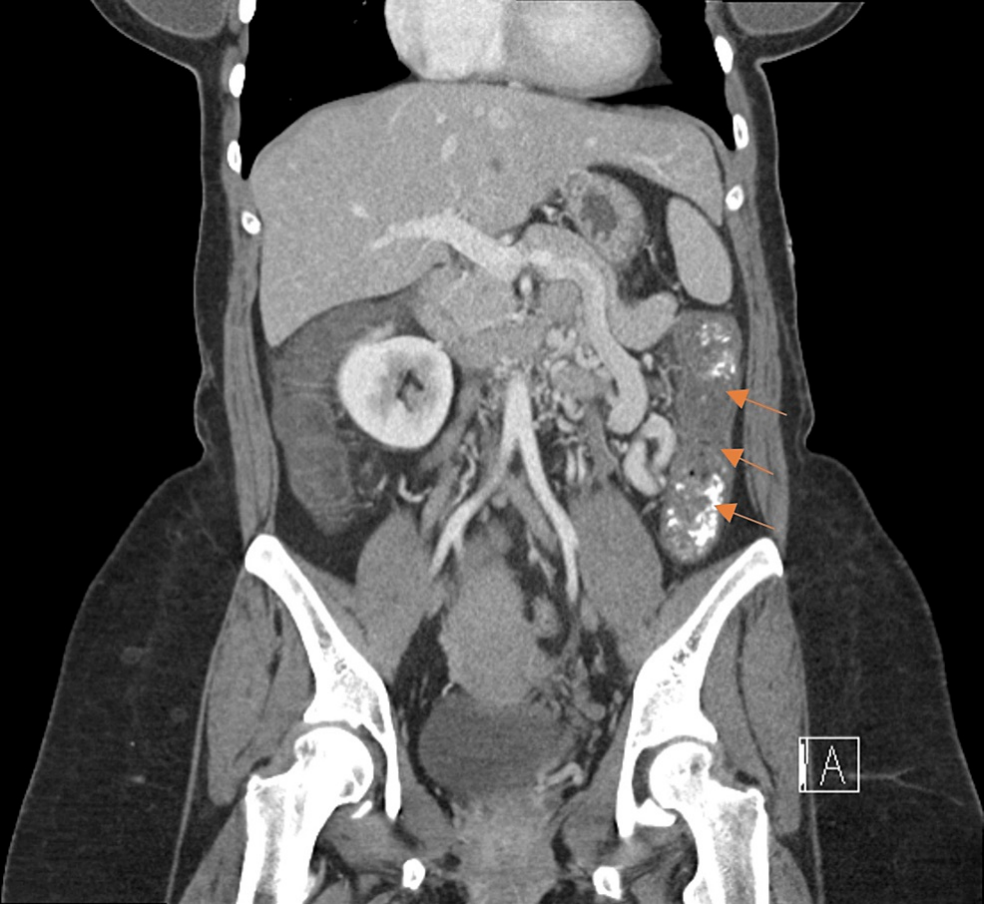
Contrast enhanced CT of the abdomen/pelvis showing diffuse thickening of the sigmoid colon (arrows), representing colitis
